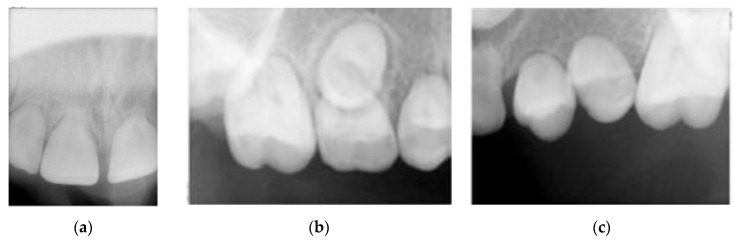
Periapical upper arch X-rays. (a) Teeth 1.1 (left) and 2.1 (right); (b) First quadrant with 1.4, 5.5 and 1.6 roots missing; (c) Second quadrant with 2.4, 2.5 and 2.6 roots missing.
